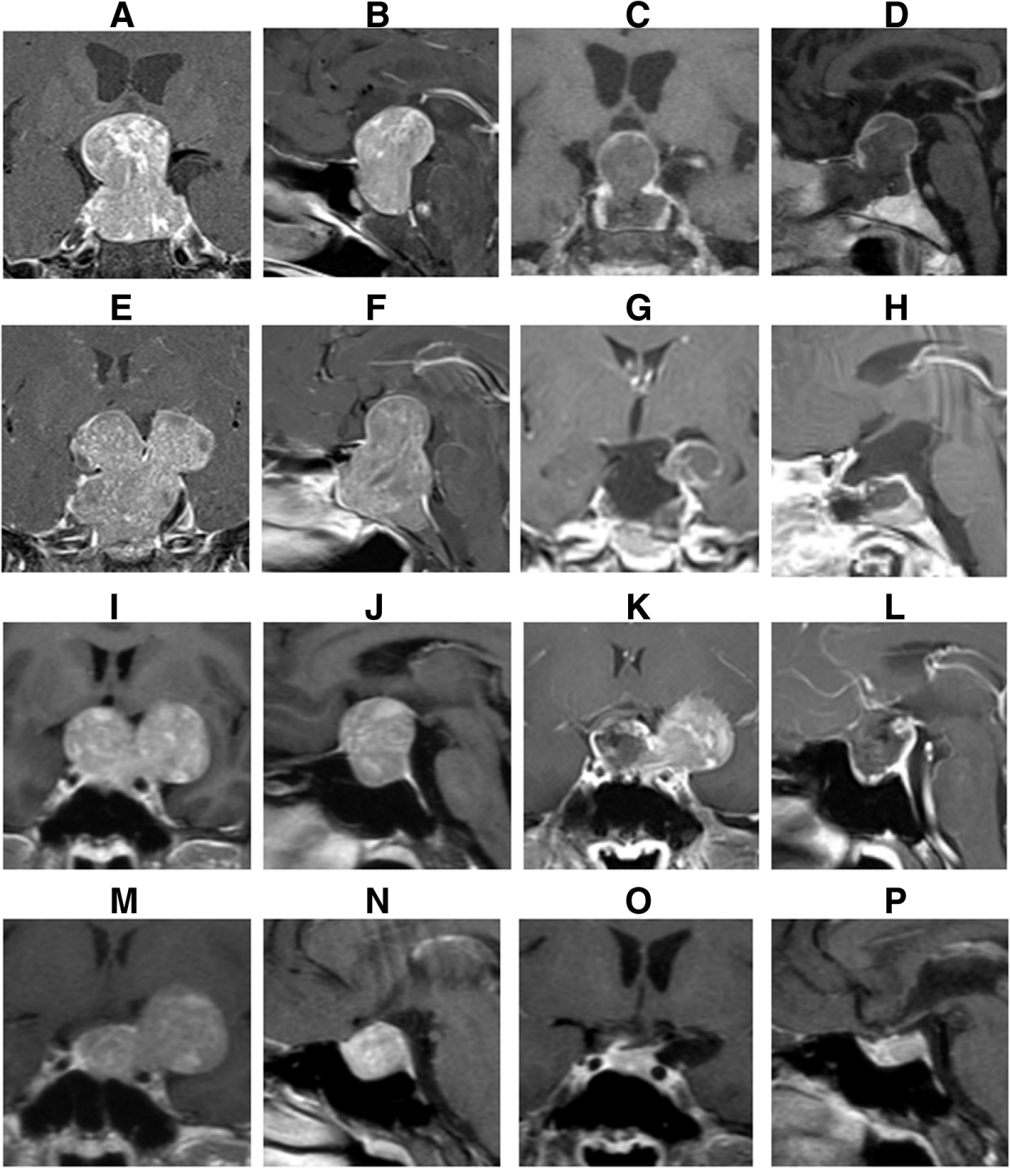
MRI of representative cases before and after surgery. (A) and (B) Coronal and sagittal MRI of Case 1 before surgery. (C) and (D) Coronal and sagittal MRI of Case 1 at 7 days after surgery. (E) and (F) Coronal and sagittal MRI of Case 2 before surgery. (G) and (H) Coronal and sagittal enhanced MRI of Case 2 at 2 days after surgery. (I) and (J) Coronal and sagittal MRI of Case 3 before surgery. (K) and (L) Coronal and sagittal enhanced MRI of case 3 at 2 days after surgery. (M) and (N) Coronal and sagittal MRI of Case 3 at 6 months after the first surgery. (O) and (P) Coronal and sagittal MRI of Case 3 at 1 year after the second surgery.
